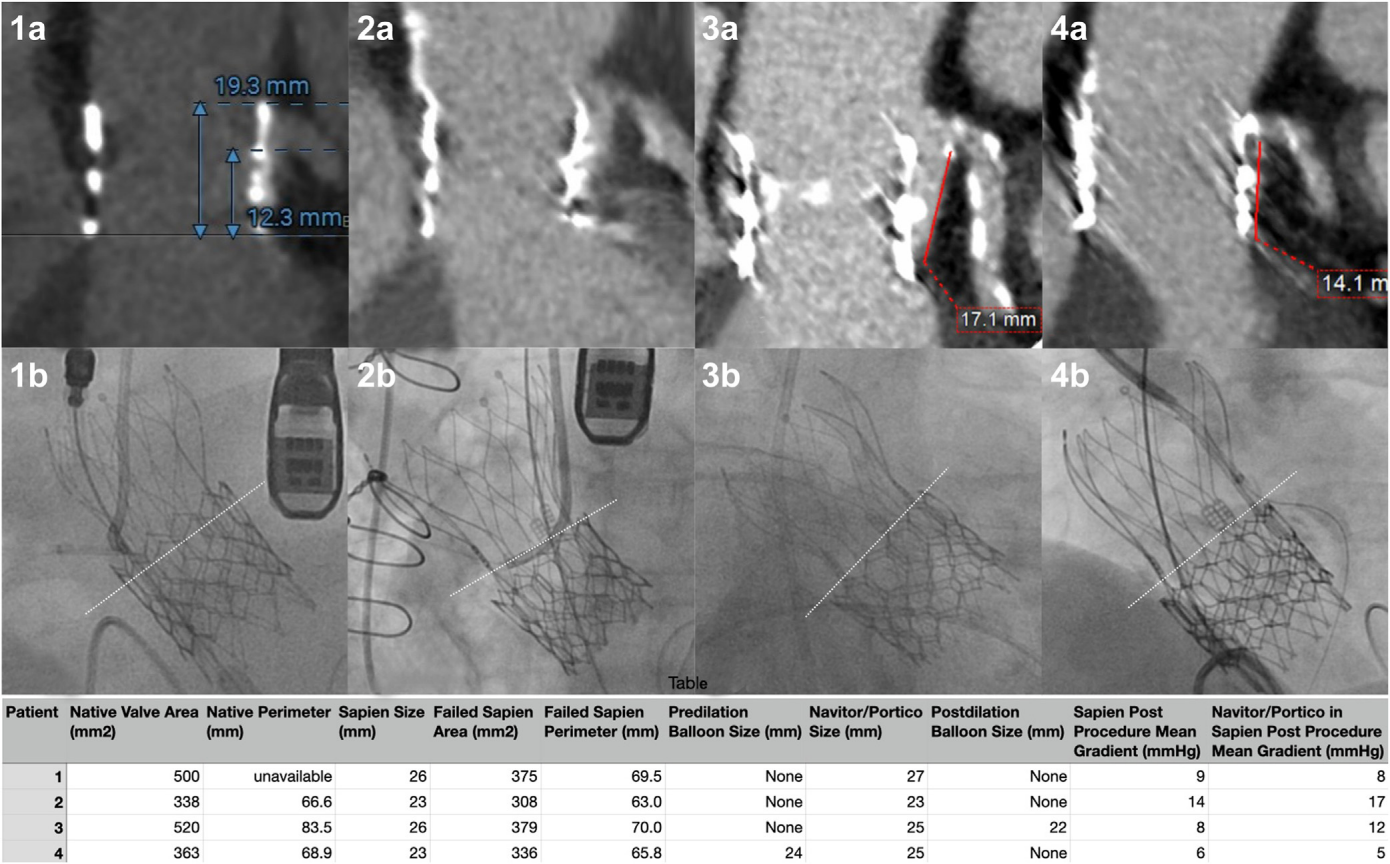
Selected preprocedural CTA images for 4 patients (1a-4a) demonstrating the relationship between the balloon-expandable TAVR frame, the sinotubular junction, and the left main coronary artery. Selected fluoroscopic images for 4 patients (1b-4b) showing the newly implanted self-expanding TAVR prosthesis within the balloon-expandable frame. The dashed line approximates the height of the newly implanted self-expanding valve leaflets. The table summarizes CTA measurements, balloon and valve sizes, and echocardiographic measurements for each patient. Abbreviations: CTA, computed tomography angiography; TAVR, transcatheter aortic valve replacement.
